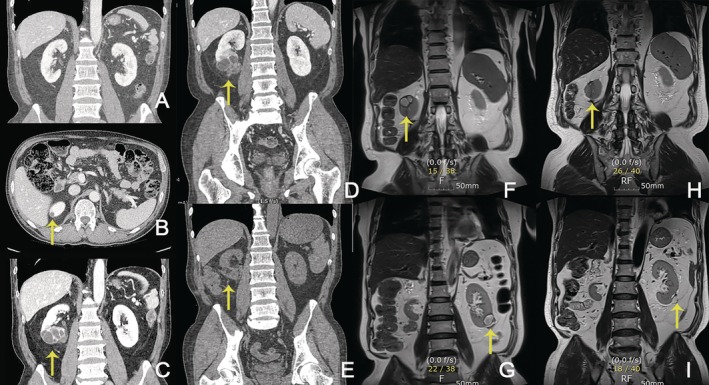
Serial abdominal computed tomography (CT) and magnetic resonance imaging (MRI) findings. (A) No renal cysts were observed 15 months after the initiation of crizotinib (August 2019). (B) A small cystic lesion in the upper pole of the right kidney was first detected on the abdominal slices of a chest CT scan 42 months after the initiation of crizotinib treatment (November 2021). (C) A newly developed complex cystic lesion in the lower pole of the right kidney, detected 49 months after the initiation of crizotinib treatment (June 2022). (D) An increase in the size of the complex renal cysts was noted 52 months after crizotinib initiation (September 2022). (E) Postoperative CT scan following partial nephrectomy (November 2022). (F, G) Enlargement of renal cysts in both kidneys (May 2024). (H, I) Complete remission of the renal cyst was observed after crizotinib dose reduction (May 2025).
